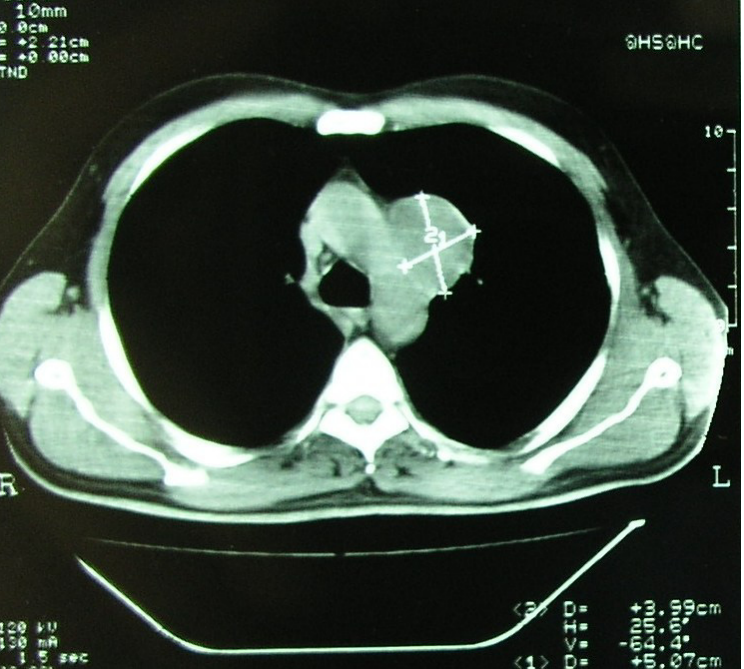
CT contrast enhanced scan of the chest showing the smooth and clear margins of the mass and its location inthe aortopulmonary window.
